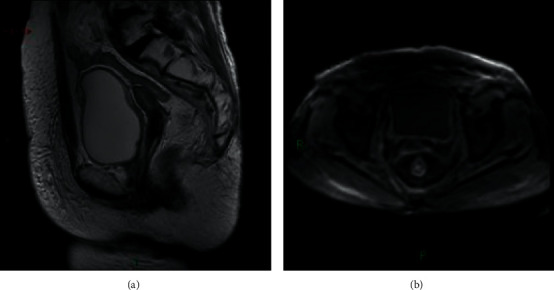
MRI findings of patients with cervical adenocarcinoma after 3 months of treatment.
